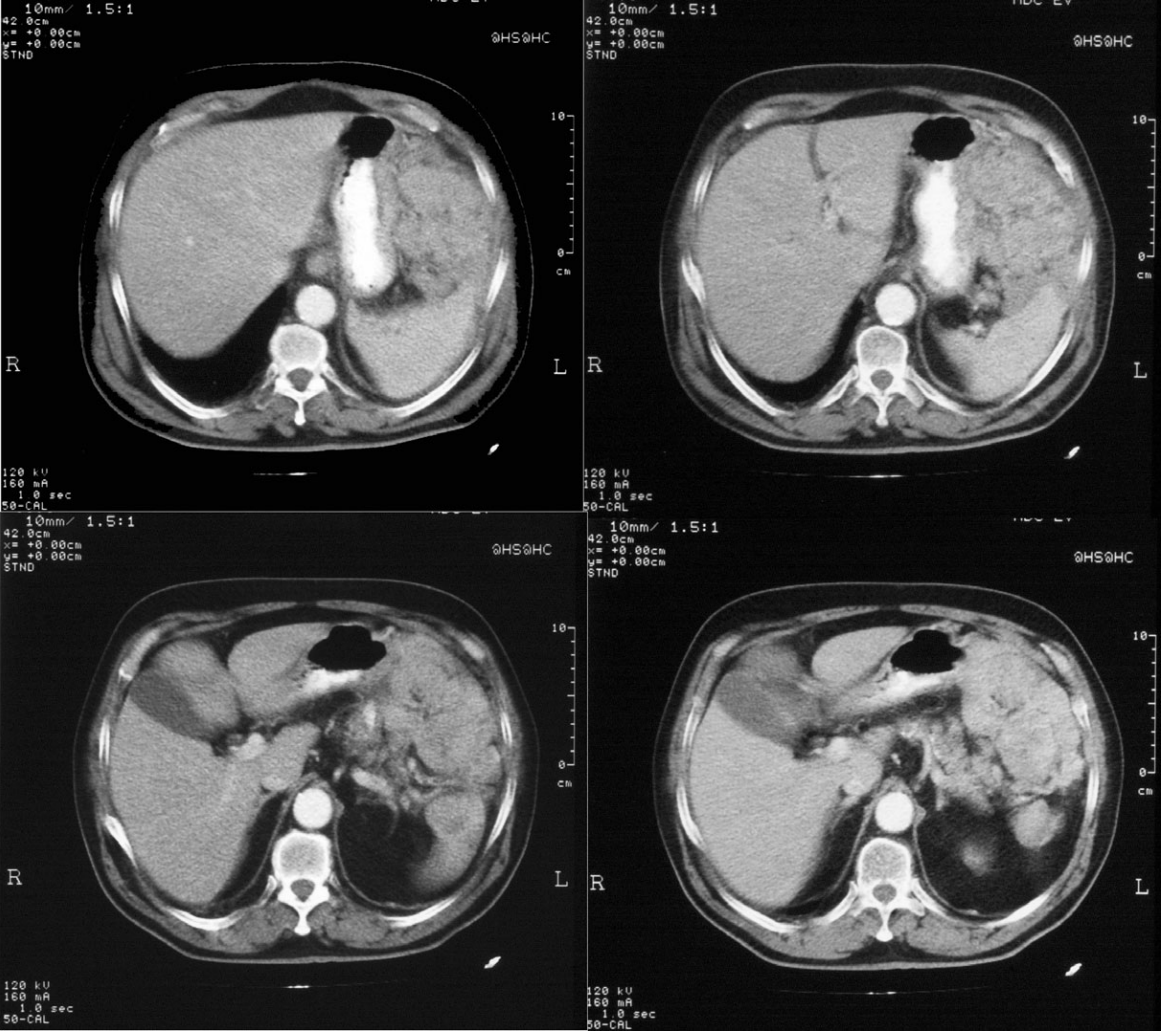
CT scan before the treatment showing the mass lesion.
